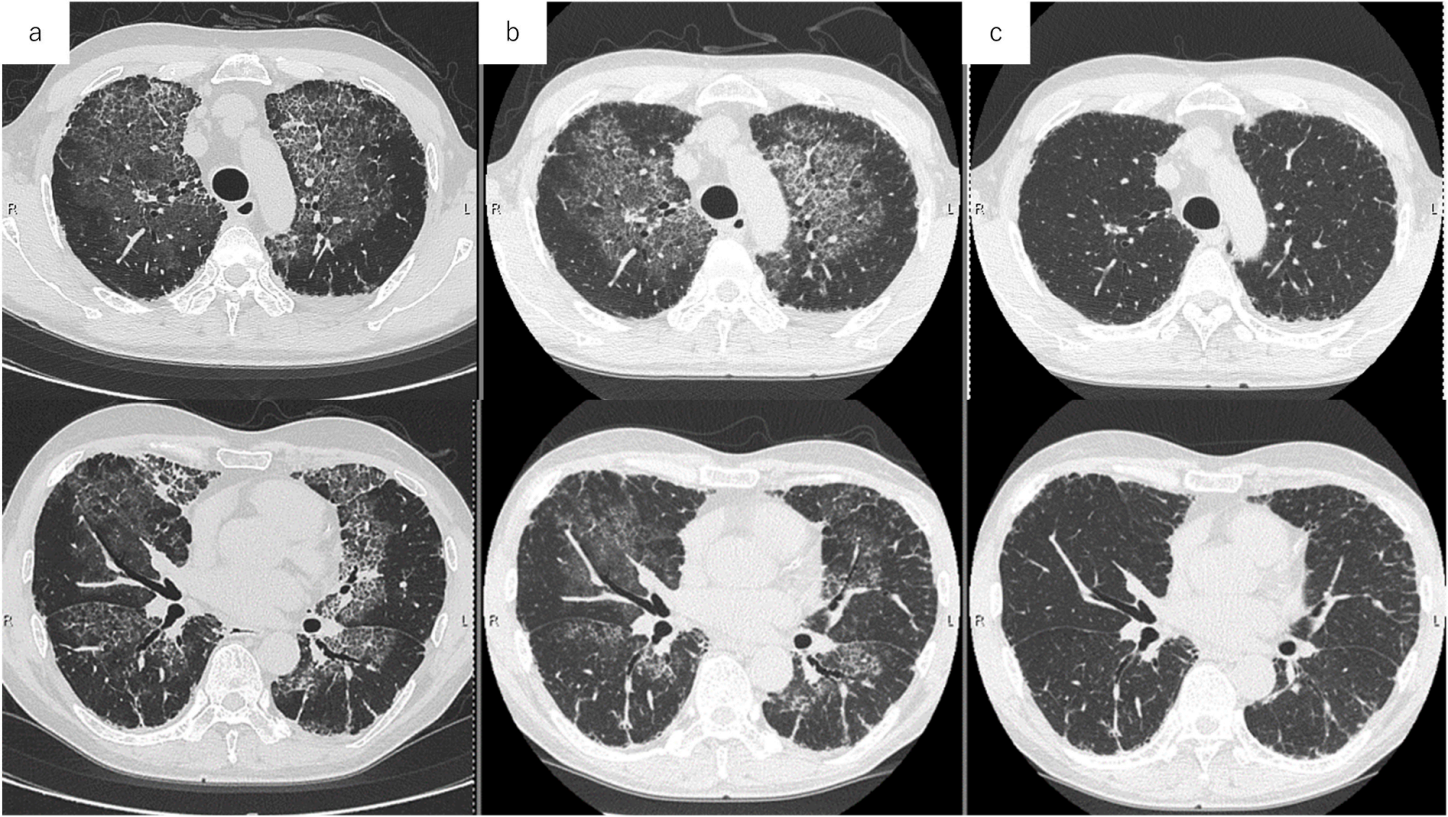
Chest CT findings in the upper and lower lung fields before and after atorvastatin administration. (a) Prior to the initiation of oral statin therapy, a diffuse crazy-paving pattern was evident in both lung fields. (b) After 8 months of oral statin treatment, improvements were observed in the ground glass and reticular changes in the bilateral lower lobes. (c) After 12 months of oral statin treatment, significant enhancements were noted in the ground glass and reticular changes throughout the entire bilateral lung field.
